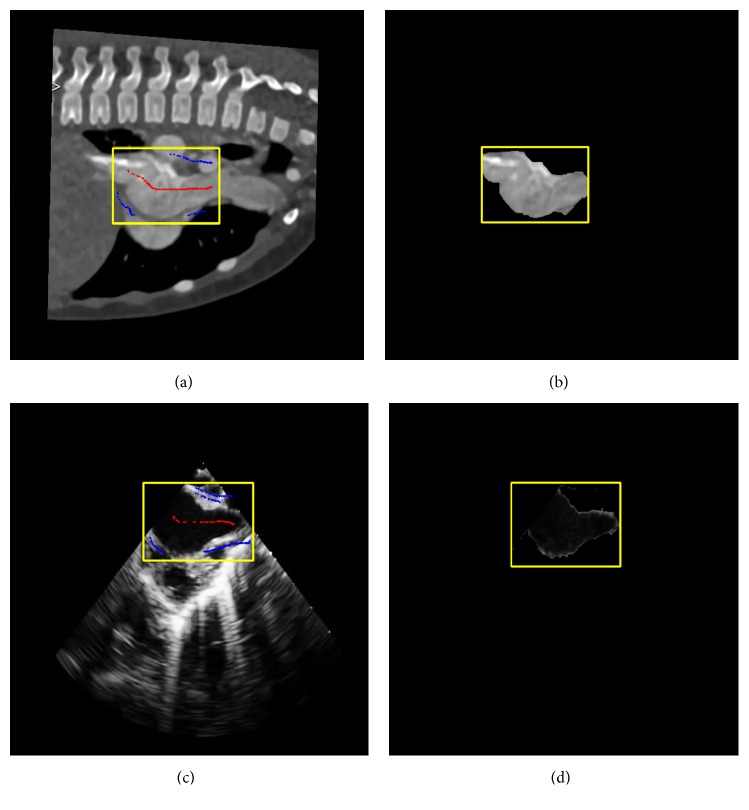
The process of interactive segmentation. (a) Interactive segmentation of a CT image. (b) The result of CT image segmentation. (c) Interactive segmentation of a TEE image. (d) The result of TEE image segmentation. Note: during interactive segmentation, the user selected a region of interest (yellow box) and marked the target (red dots) and the background areas (blue dots). CT, computed tomography; TEE, transesophageal echocardiography.
